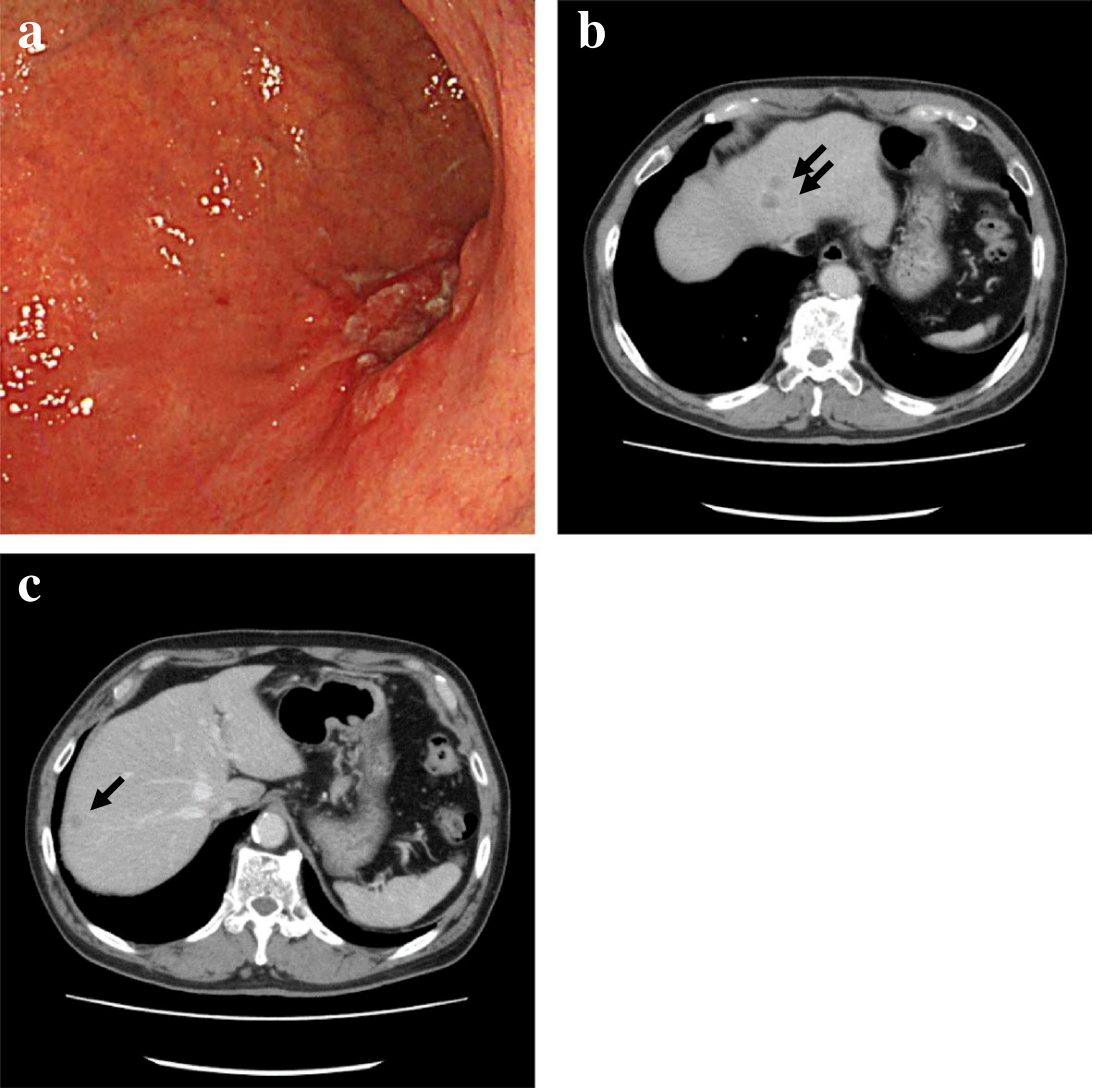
Gastrointestinal endoscopy and computed tomography findings at the time of diagnosis of gastric cancer. a Endoscopic view of advanced gastric cancer on the posterior wall of the lower part of his stomach. b, c Liver metastases (*arrows*) detected by computed tomography
